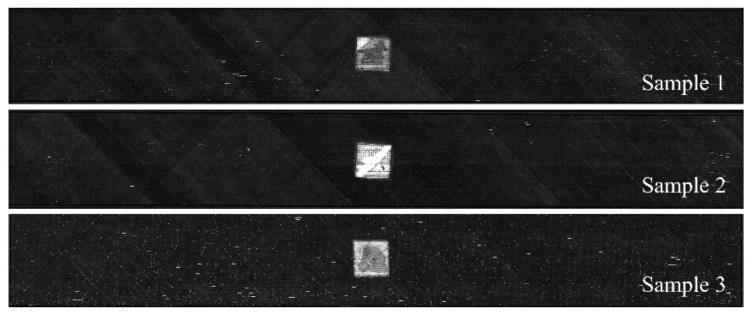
Ultrasonic scanning results of samples with delamination.
